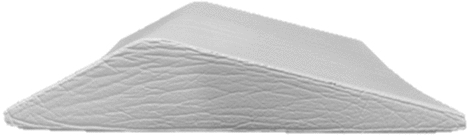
picture of the bolster used in the MRI scan to provide extension of the spine. The bolster is 9 cm height, 40 cm large and 45 cm in depth
